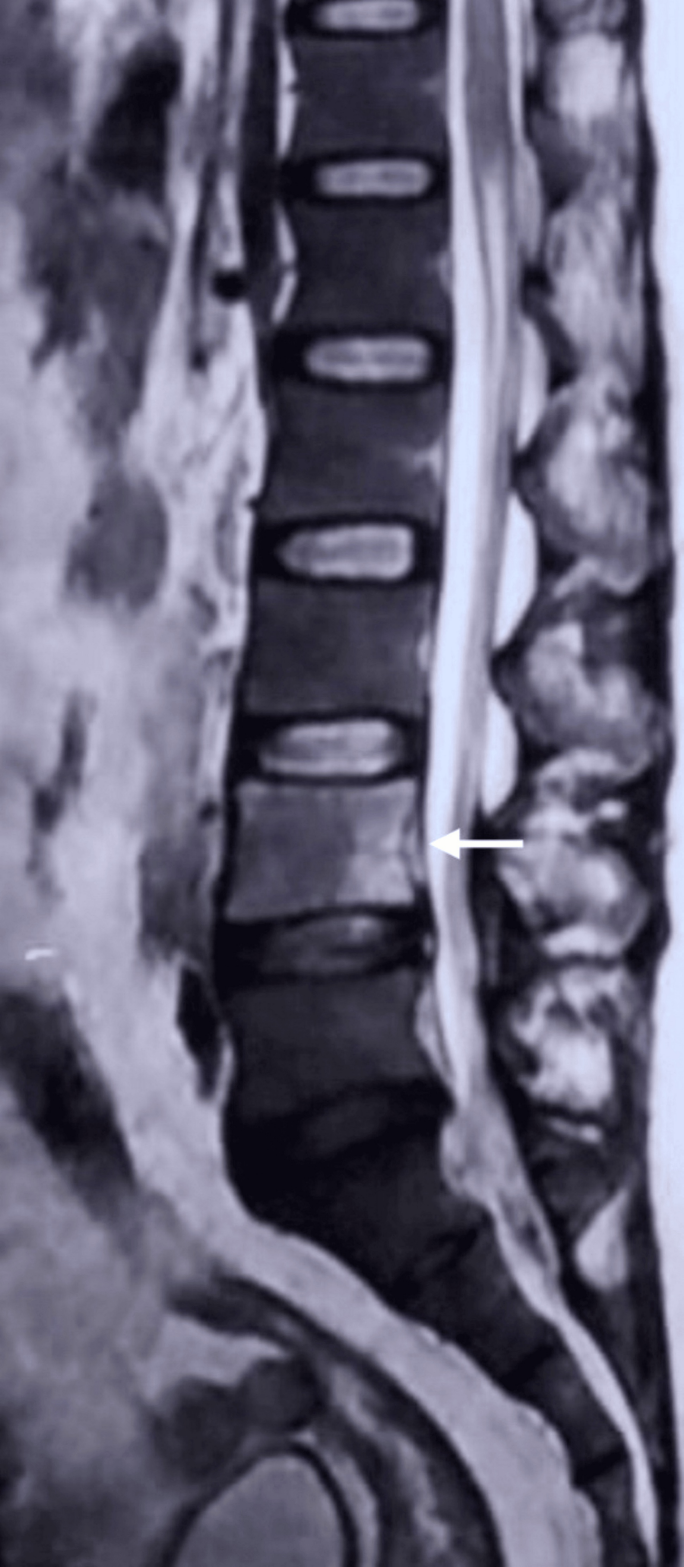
MRI of the lumbosacral spine demonstrating destructive vertebral changes centred at L4. Sagittal MRI image of the lumbosacral spine showed destructive vertebral changes at the L4 level with adjacent inflammatory soft-tissue change. The arrow highlights the L4 vertebral body level corresponding to the site of maximal abnormality, in keeping with spinal infection in the clinical context.
